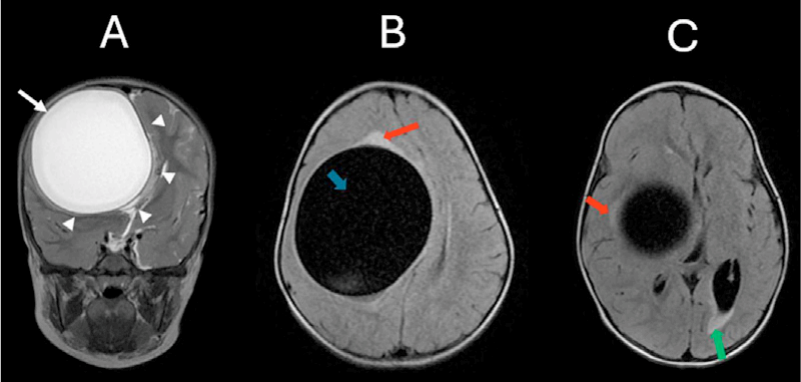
(A) Coronal T2-weighted MRI demonstrates a large, well-defined intra-axial cystic lesion in the right frontoparietal region with homogeneously hyperintense signal content (white arrow). The lesion exerts significant mass effect on the midline structures (white arrowheads). (B & C). Axial T2 FLAIR images show complete suppression of the cystic fluid signal within the lesion (blue arrow). A faint hyperintense pericystic rim is noted (red arrow), suggestive of minimal surrounding edema. Image C additionally reveals periventricular FLAIR hyperintensity, most pronounced along the left occipital horn (green arrow).
